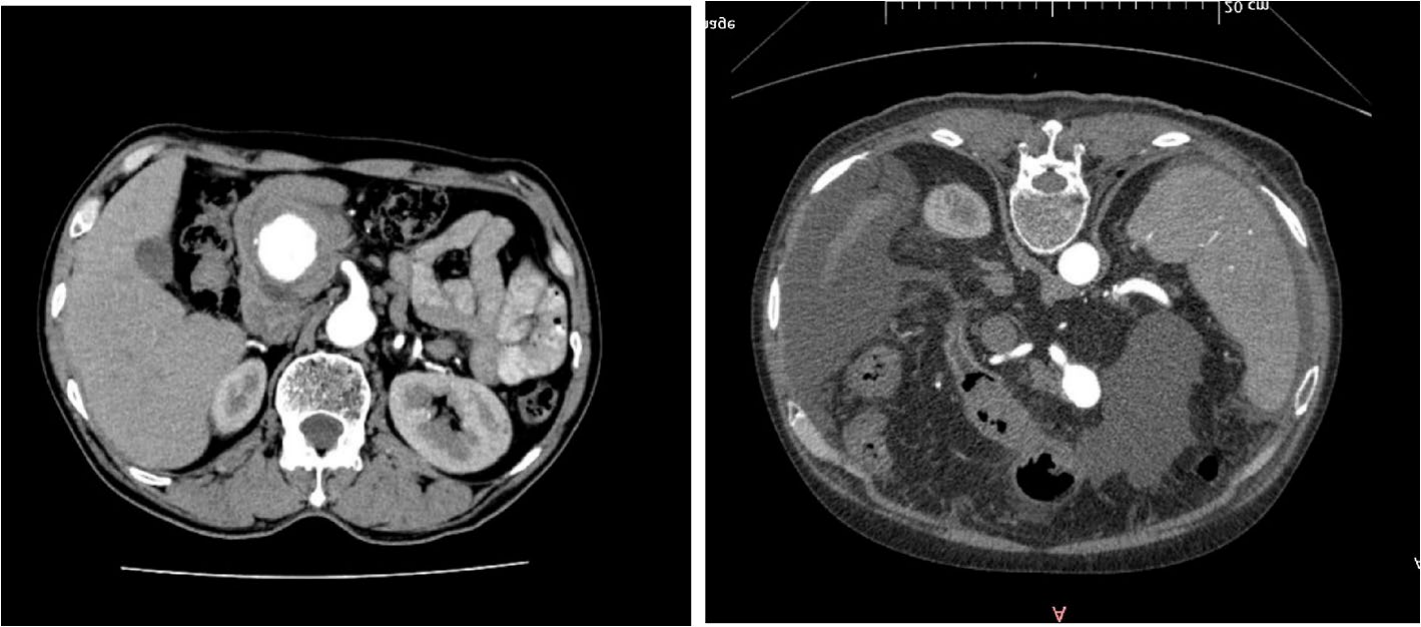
(A) CT imaging of pseudoaneurysm. (B) CT imaging of true aneurysm.
